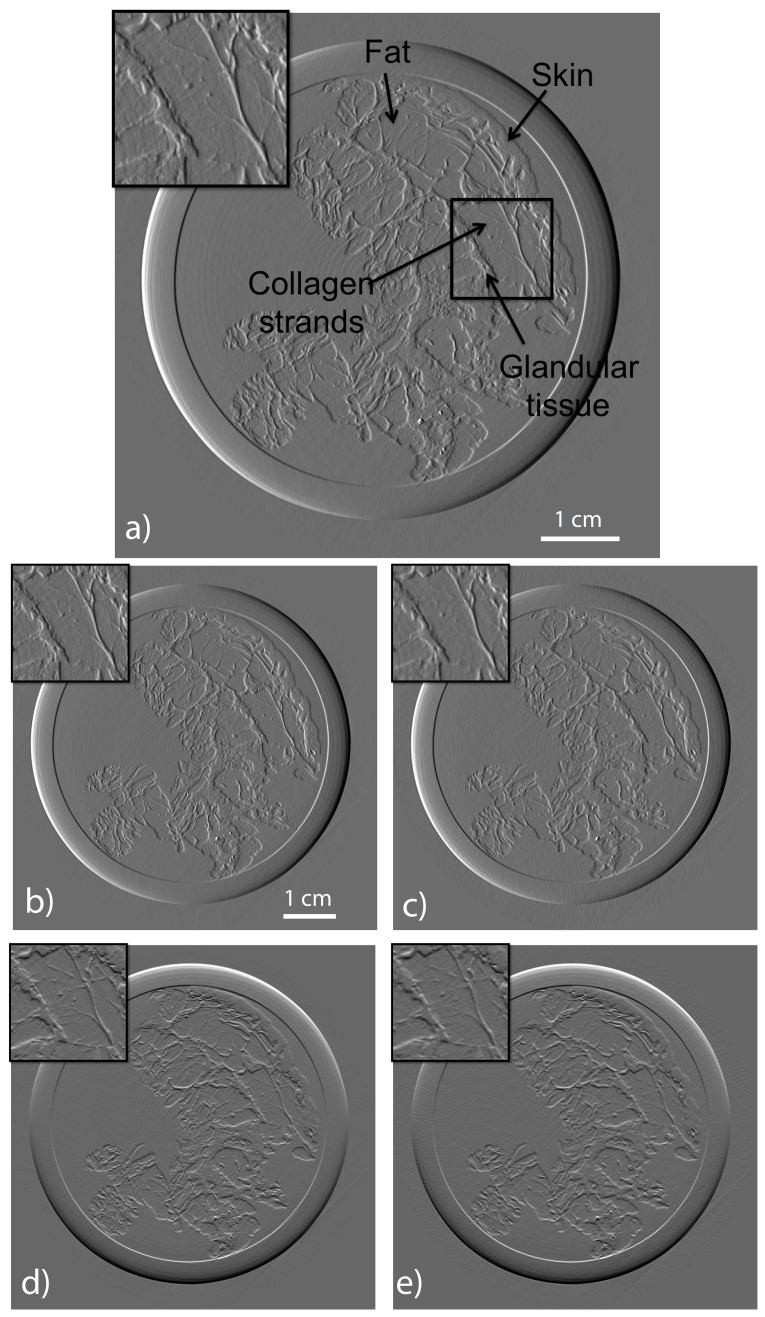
Reconstruction of a computed tomographic slice of the breast. The images on the first and second row are the X and Y phase gradients, respectively. In the left column the results of the reconstruction obtained with the FBP method using the full set of data are reported. In the right column the results of our method using one projection over five are shown. For these reconstructions we set
